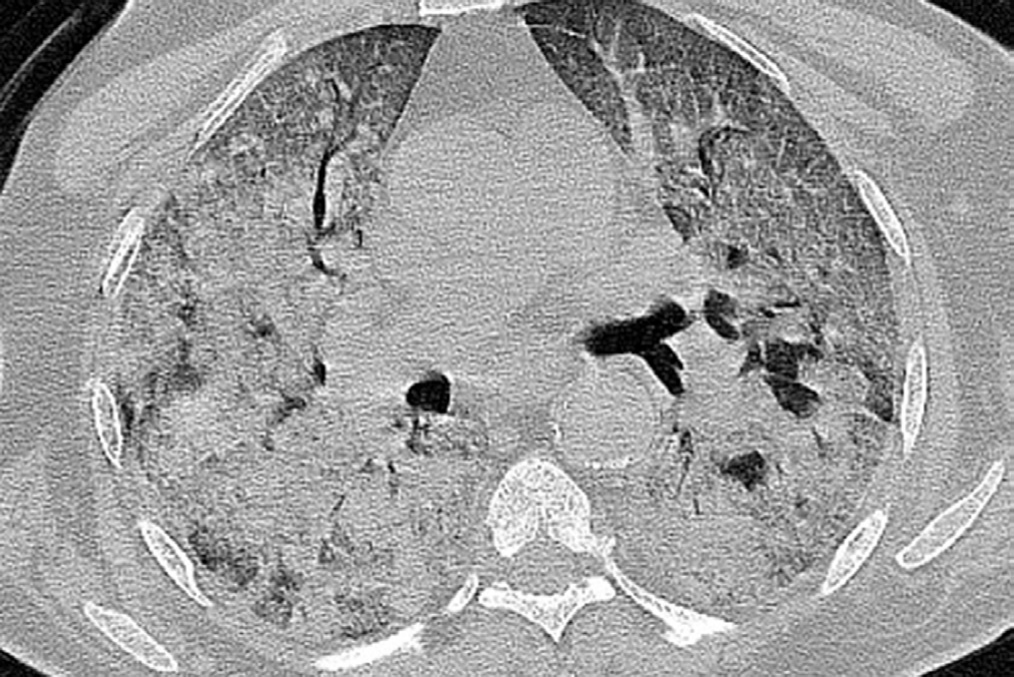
Axial CT image of a 68-year-old patient with COVID-19 pneumonia (δ variant), with symptoms for 4 days, showed bilateral extensive consolidations. The patient died 5 days later.
